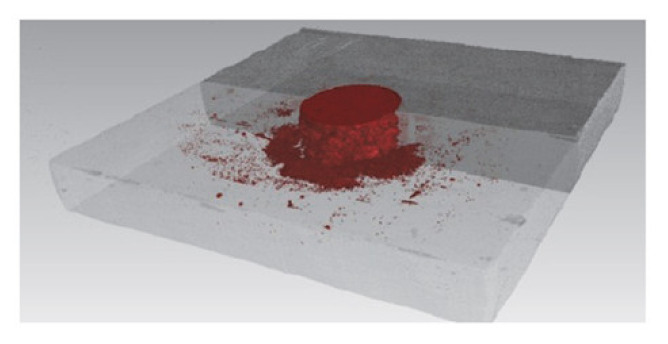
Volumetric computed tomography scan of delaminations around a punched hole in carbon fibre reinforced plastic during SPR [29].
